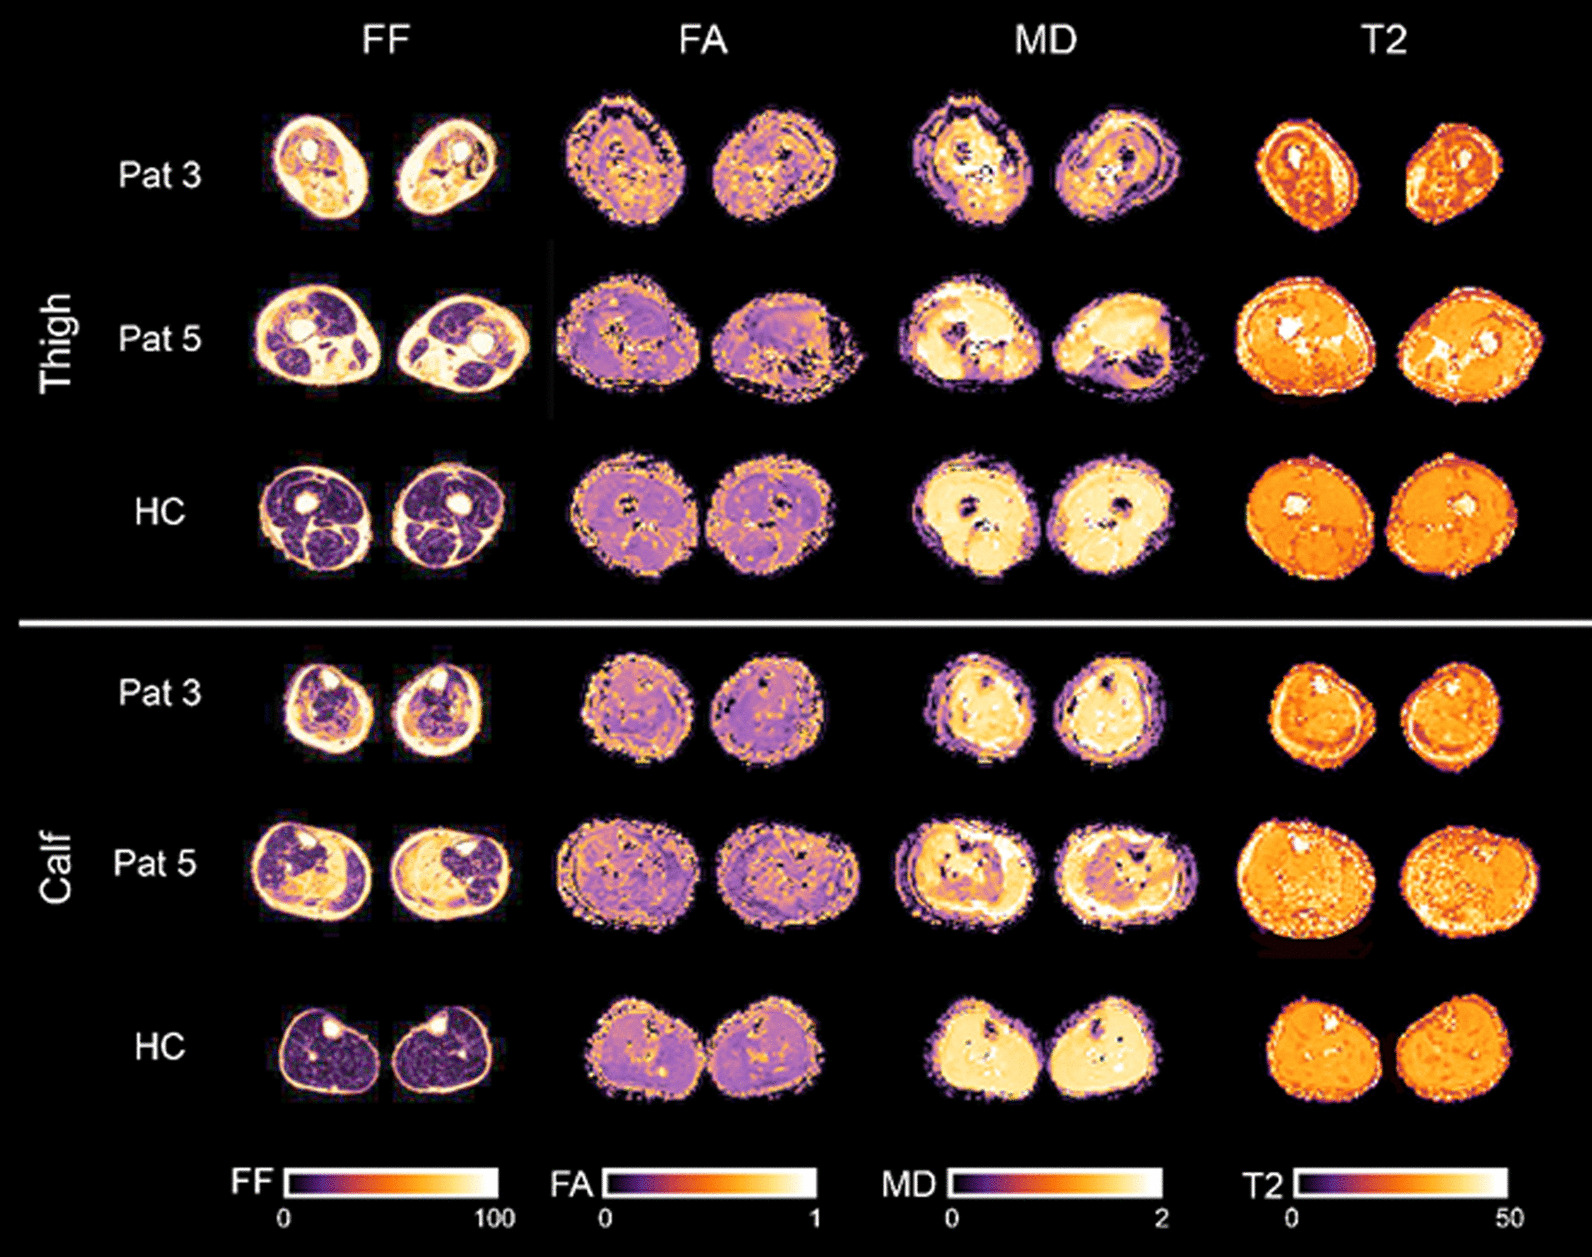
Example images of the applied MRI sequences: mDixon fat fraction (FF), fractional anisotropy (FA), mean diffusivity (MD) and water T2 maps for thigh and calf muscles of two representative patients, and a healthy control (HC)
